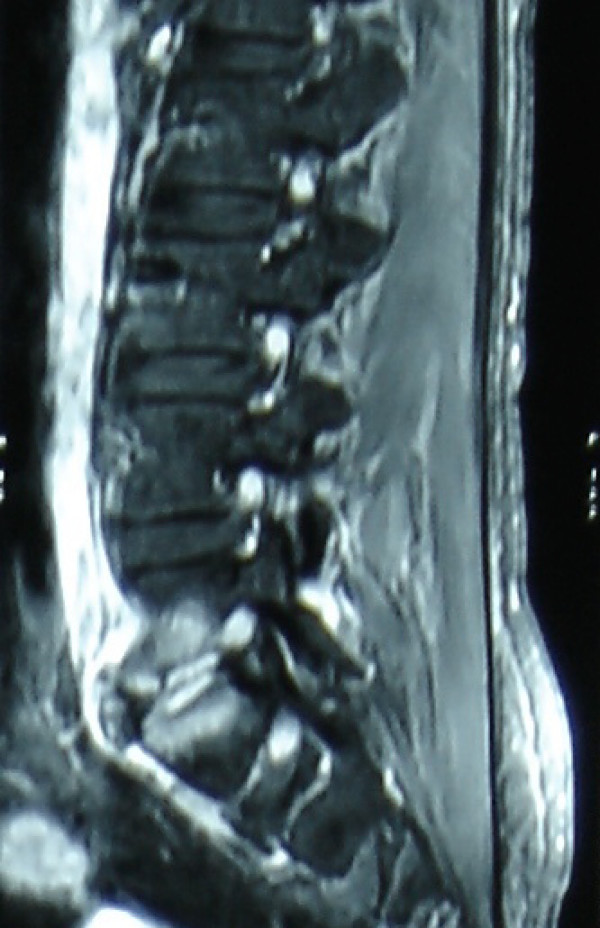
MRI (STIR sequence) showing hyperintense signal from L_5 _and S_1 _vertebrae and the intervertebral disc suggestive of acute infective discitis.
